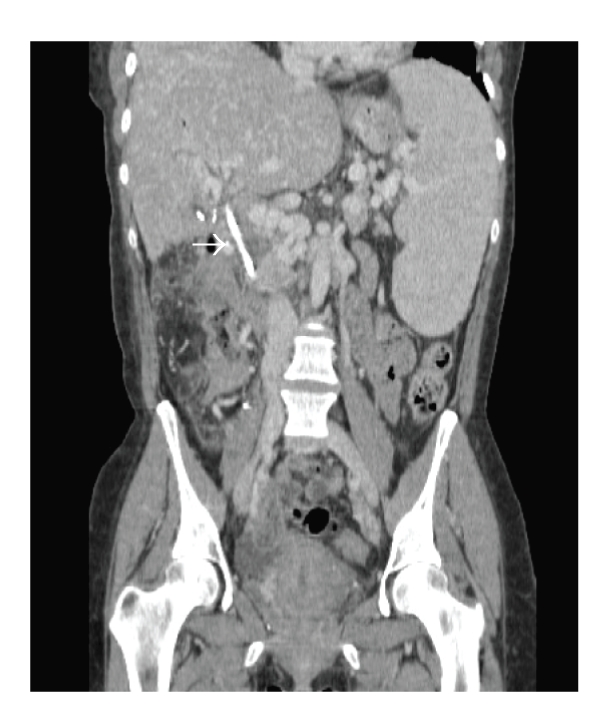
Postoperative coronal abdominal contrast enhanced CT scan shows opacification of the mesogonadal shunt (white arrow) confirming its patency.
